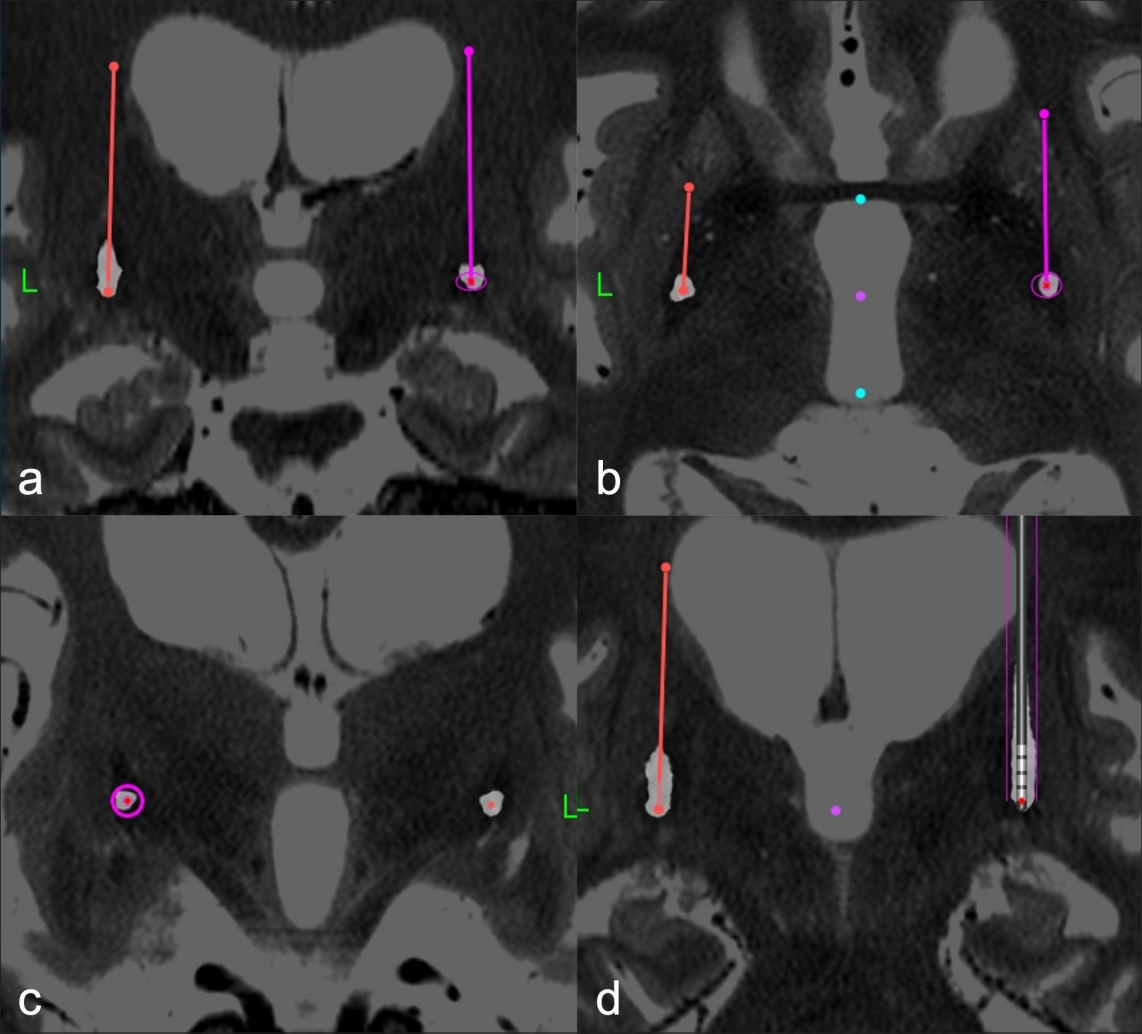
A 3-moths postoperative CT was co-registered with a T2 sequence preoperative MRI (StealthStation 7, Cranial stereotactic software, Medtronic). Coronal (a), axial (b), and probe’s eye (c) views of the definitive location of the DBS electrodes, (d) shows a parallel reconstruction of the right DBS electrode (Medtronic 3389). The tips of both electrodes were located in the posterolateral region of the ventral GPi, close to the interpallidal lamina. The definitive location coordinates of the electrodes were as follows: rGPi X = 24.7 mm, Y = 1.4 mm, Z = –0.8 mm; lGPi X = 24.5 mm, Y = 1.4 mm, Z = –0.3 mm.
